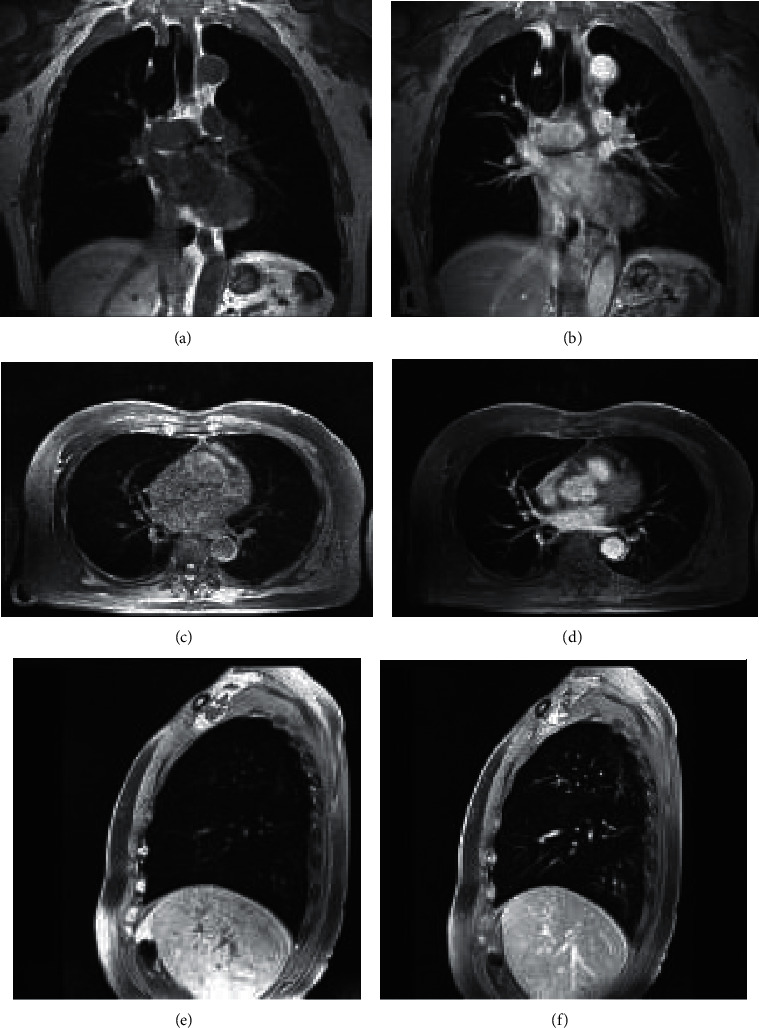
MRI results. (a) Not enhanced coronal. (b) Enhanced coronal. (c) Not enhanced axial. (d) Enhanced axial. (e) Not enhanced sagittal. (f) Enhanced sagittal. Note: male patient's tumors in the posterior segment of the left lung are clearly displayed. Nonvascular branches and bronchi in the lung parenchyma are normally displayed without vascular interference.
